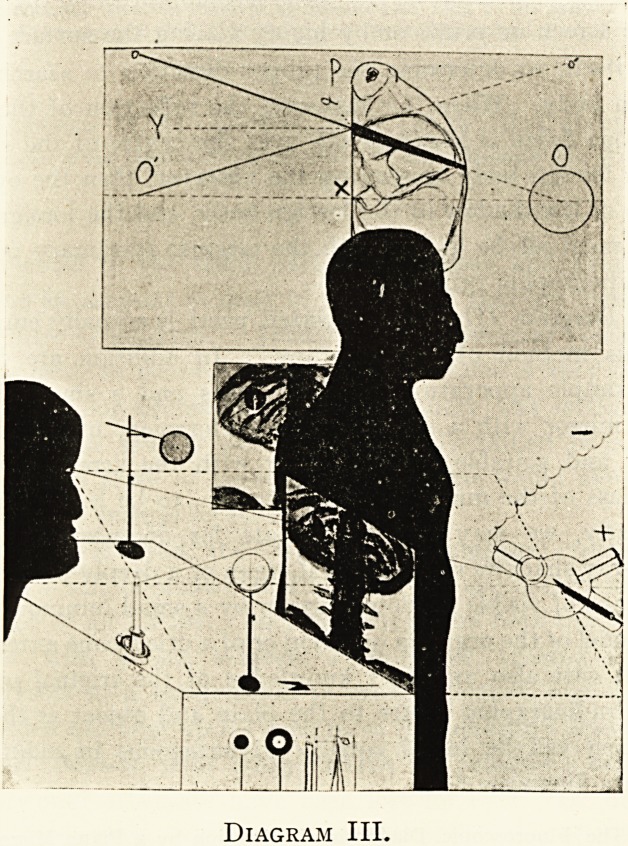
# Design for an Elementary Radiographic Camera
